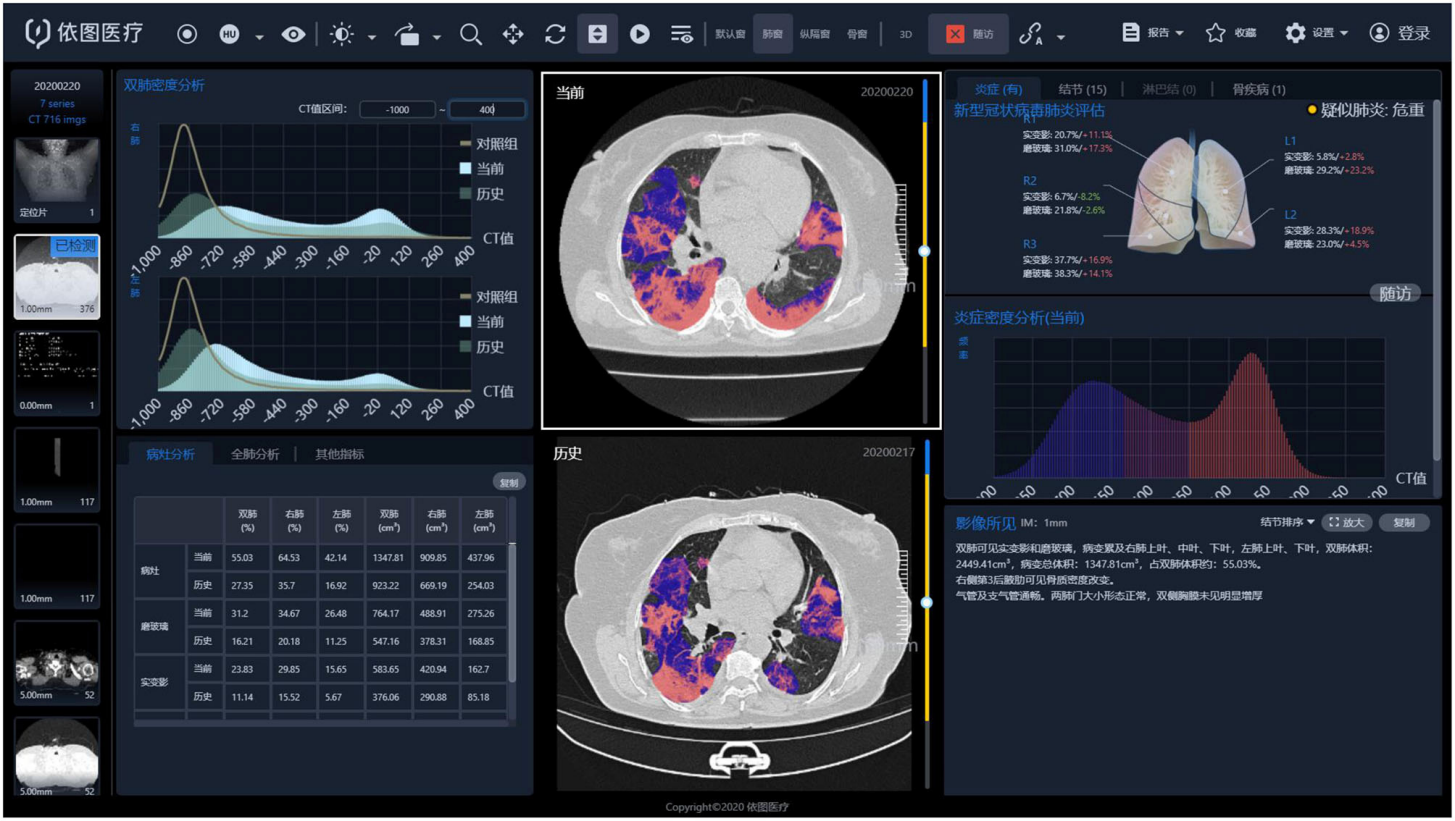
CT image quantization and analysis with artificial intelligence system.
